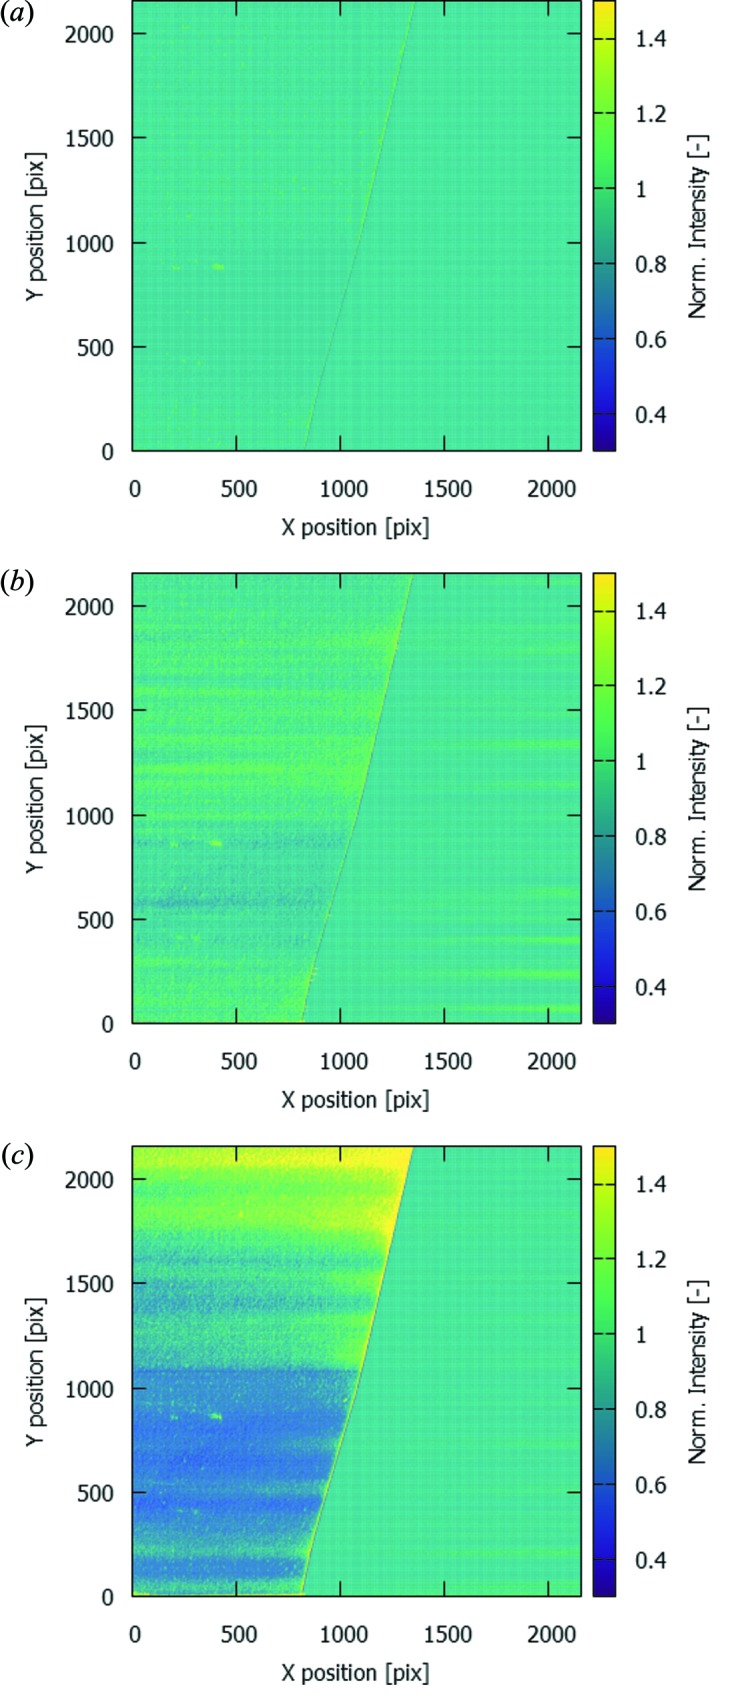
Normalized radiographic images of a 10 µm-thick gold foil (on the left in each image) using (*a*) pink beam, (*b*) MLM monochromator below the Au *L*
